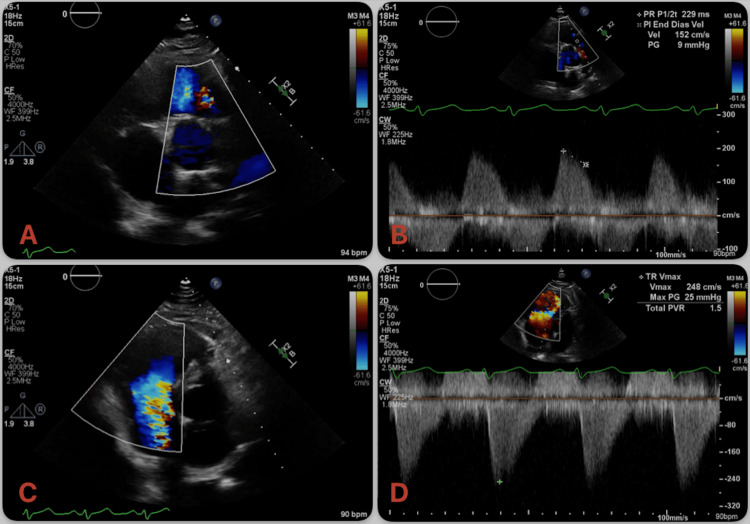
Transthoracic echocardiography (TTE) demonstrating carcinoid heart disease with dual right-sided valve involvement TTE demonstrating (A) severe pulmonic valve insufficiency (PI) in short-axis view, (B) with Doppler profile consistent with severe PI, and (C) restricted motion of the tricuspid leaflets with no coaptation and wide torrential tricuspid regurgitation, with (D) dagger-shaped Doppler profile, consistent with carcinoid heart disease.
